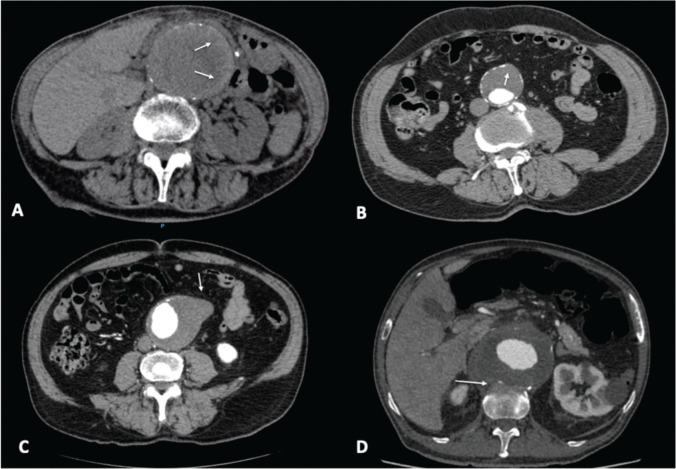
Axial abdominal CT images showing infrarenal abdominal aortic aneurysms with SIR (white arrows). A: Crescent sign. B: Focal wall discontinuity of circumferential calcifications. C: Aortic bulges or blebs. D: Draped aorta sign.
